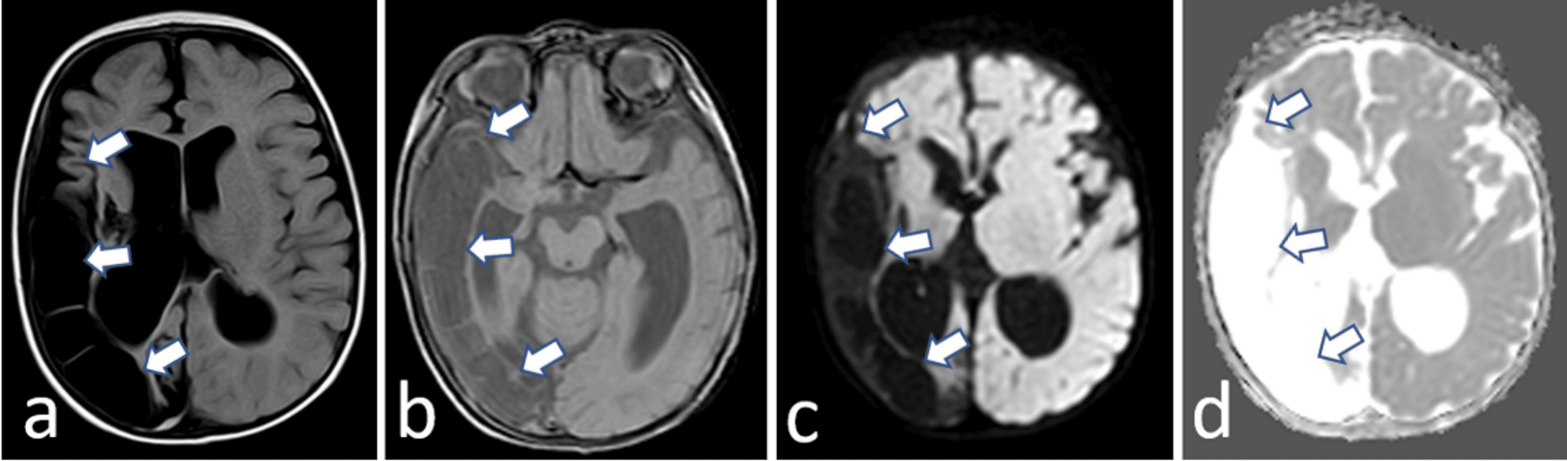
Brain MRI axial sequences. Fluid-attenuated inversion recovery (FLAIR) (a), SET1 (b), diffusion b1000 (c), and apparent diffusion coefficient (ADC) mapping (d). White arrows indicate a large right temporo-parieto-occipital area hypointense in FLAIR, T1, and diffusion, without diffusion restriction on ADC mapping. These findings are consistent with chronic stroke with resulting encephalomacia.
